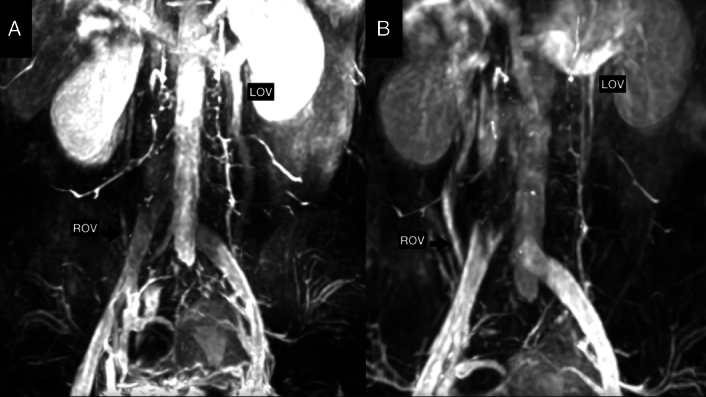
A female patient with pelvic congestion syndrome: Her chronic pelvic pain has a poor response to medication. A. Her engorged left ovarian vein was visualized (LOV). The caudal flow of the left ovarian vein was confirmed by quantitative 2D PC MRI. A small right ovarian vein could also be seen (black arrow). B. Her pelvic pain disappeared after left ovarian venous embolization. However, the pelvic symptoms recurred 3 years later. The second Quantitative 2D PC MRI showed the atrophic left ovarian vein (LOV), with a larger right ovarian vein (black arrow) than 3 years ago.
